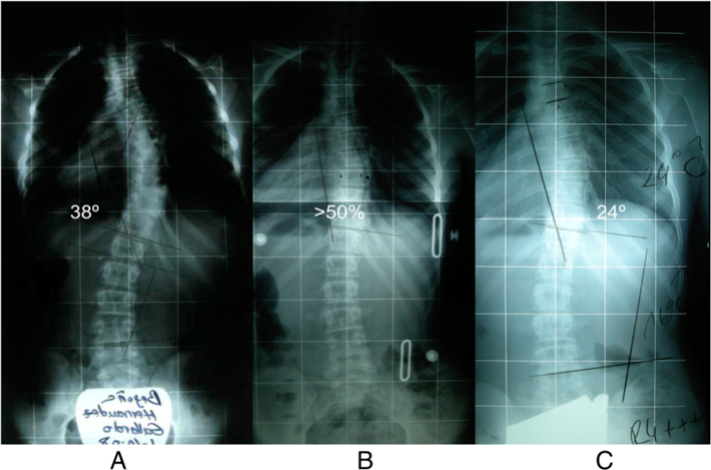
(a, b, c): Series of radiographs of a patient with progressive scoliosis treated with the Rigo-Chêneau brace. a The initial radiograph shows a thoracic scoliosis curve of 38° Cobb. b In-brace radiograph shows a greater than 50 % scoliosis curve correction. c Out-of-brace radiograph at Risser 4 (end of growth) shows that the thoracic curve has been decreased significantly to 24° Cobb angle, a curve reduction of >35 % compared to the initial radiograph
